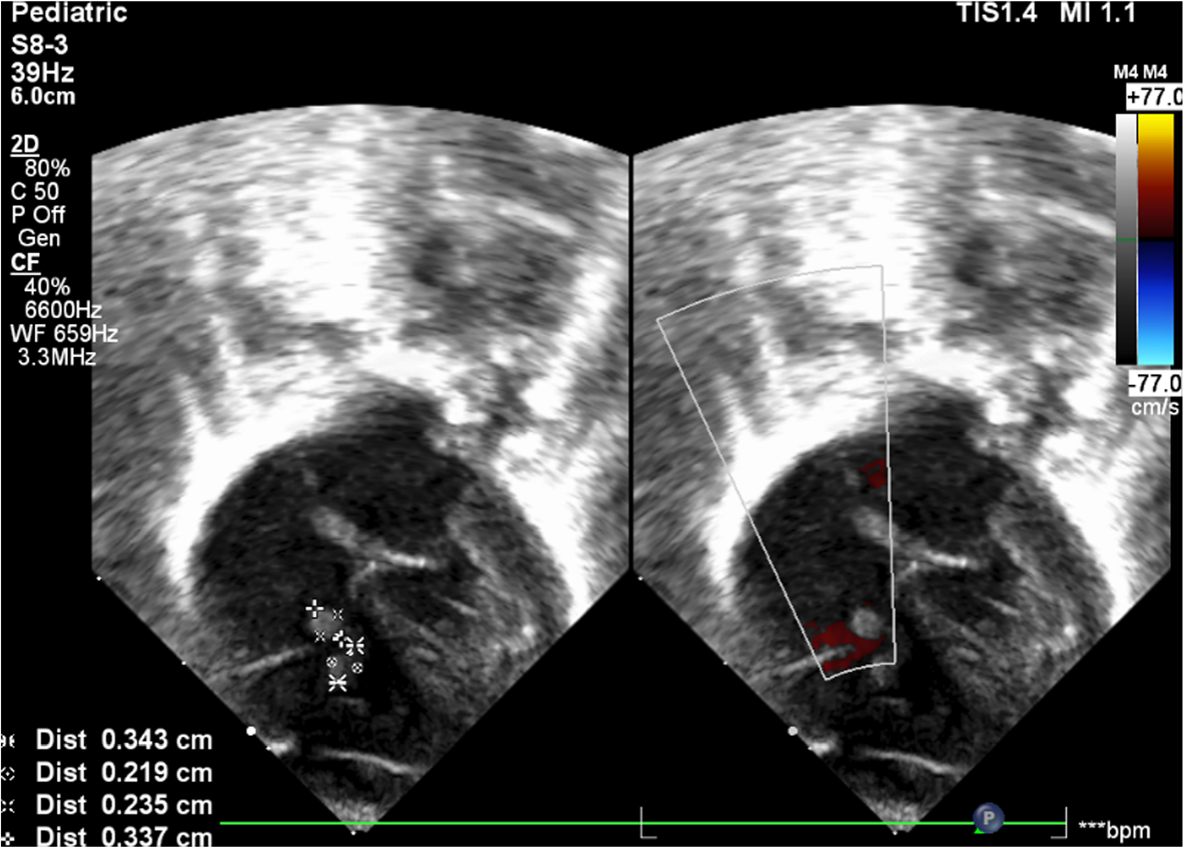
Echocardiography showing multiple vegetations
